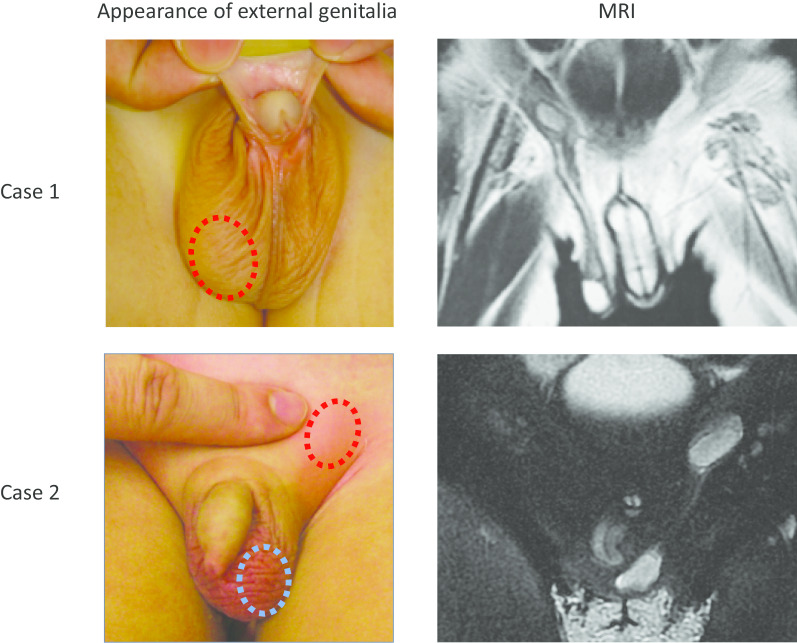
Appearance of external genitalia and MRI findings in cases 1 and 2. Dotted circles indicate palpable testes
